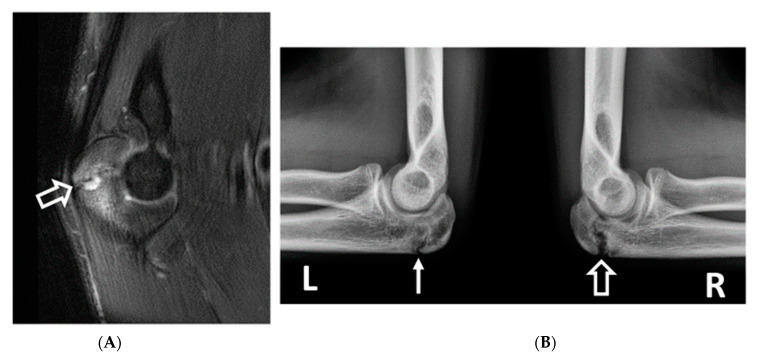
A 15-year-old right-hand-dominant male baseball pitcher with right posterior elbow pain. (A) Sagittal fat-saturated T2-weighted image of the right elbow demonstrates irregularity, increased T2 signal and cystic change along the olecranon physis (open arrow), with periphyseal bone marrow edema, compatible with olecranon apophysitis. (B) Radiographic comparison of the bilateral elbows demonstrates physeal widening, irregularity, and delayed closure of the physis (open arrow) compared with the physis of the asymptomatic left elbow (arrow), which is partially fused superiorly.
